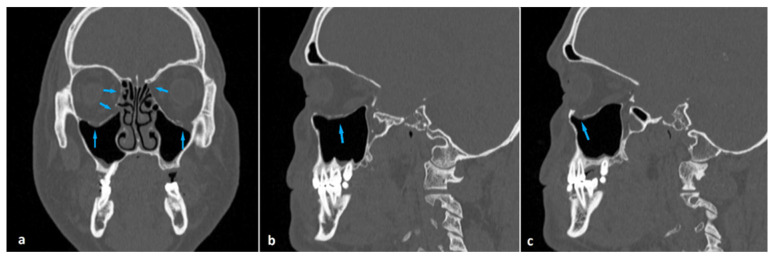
Coronal (a) and sagittal (b,c) maxillofacial computed tomography images of a 57-year-old man presenting with head trauma. He had bilateral blowout fractures (arrows) of the medial orbital walls and orbital floors (a). Right and left orbital floor fractures with herniation of fatty tissue into the maxillary sinuses (arrows) are shown in (b) and (c), respectively.
